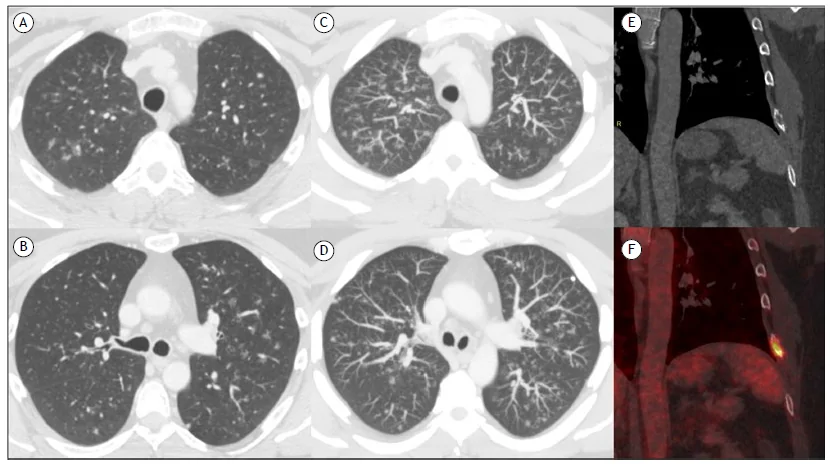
In A and B, axial chest CT images at the upper and middle lung zones demonstrate multiple relatively well-circumscribed nodular opacities, predominantly distributed along the peribronchovascular interstitium, with variable attenuation ranging from ground-glass to mildly solid components. In C and D, axial maximum-intensity-projection (MIP) reconstructions highlight the extent and peribronchovascular predominance of these nodular opacities. In E and F, CT and fused PET/CT images show an aggressive osteolytic lesion of the left eighth rib, with cortical breakthrough and marked hypermetabolic activity (SUVmax = 8.7). In G and H, CT and fused PET/CT images reveal a lytic lesion in the right intertrochanteric femur characterized by internal septations, foci of mineralized matrix, and a peripheral sclerotic rim, demonstrating moderate radiotracer uptake (SUVmax = 5.8) without cortical destruction.
